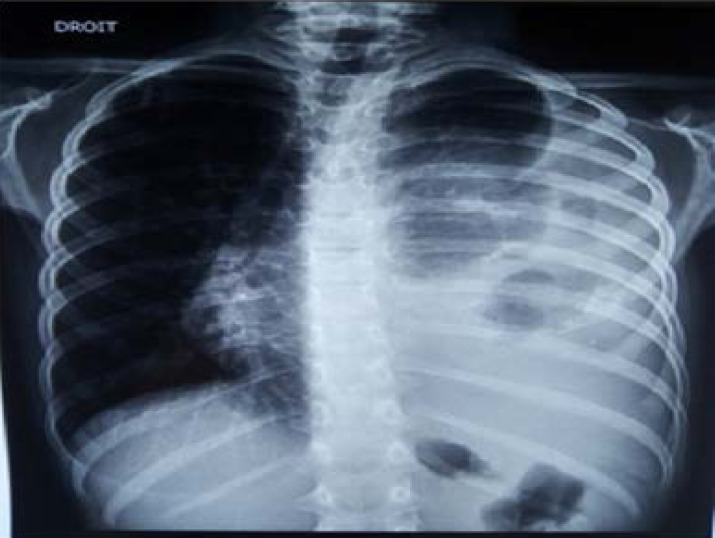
Chest X-Ray (P-A view) showing high density opacities occupying two-thirds of the left lung with cardiomediastinal shift to the right.
